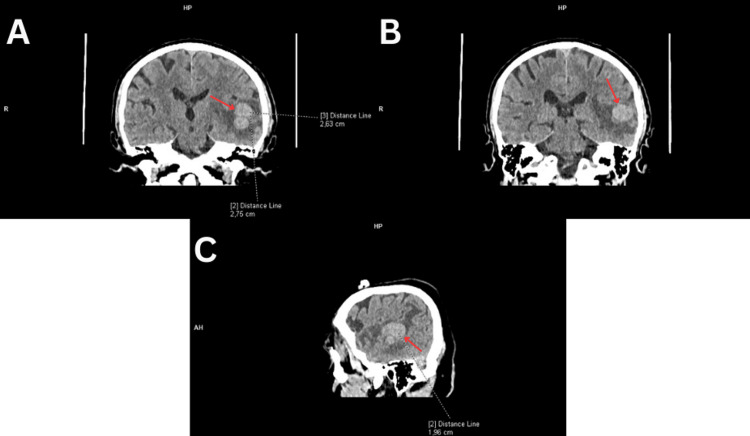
A-C. Head computed tomography without contrast. A faint heterogeneous mass in the left temporal lobe (red arrows) is seen with midline shift.
